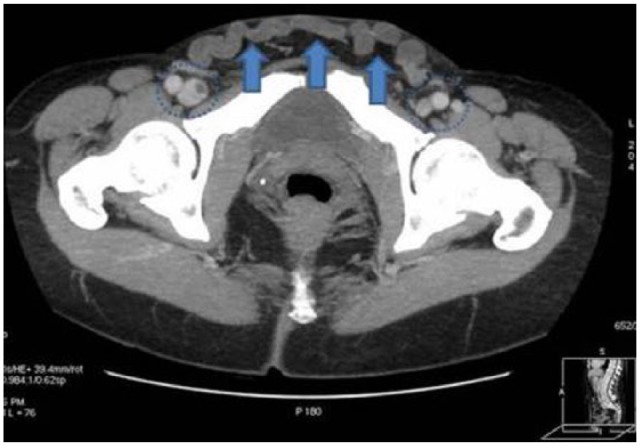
Axial maximum intensity projection (MIP) CT image showing the serpentiform varicose vein connecting the two common femoral veins (circle marker). The venous collateral appears unspecified due to the presence of thrombus which is also seen freely floating inside the right common femoral vein (arrowhead).
